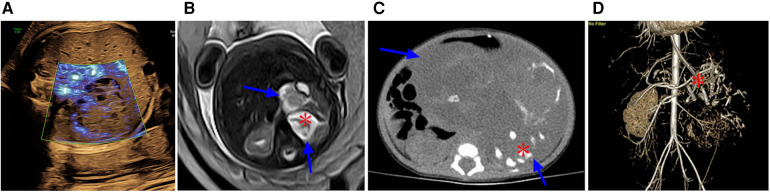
(A) At 29 weeks of gestation, a solid cystic mass with a clear boundary was observed in front of the fetal left kidney. Prenatal ultrasound indicated an adrenal solid cystic mass. (B) Image obtained at 29 weeks of gestation showing a parasitic fetus in the left upper abdomen. T2WI in the transverse view showed two interconnecting lesions in the left upper abdomen (blue arrow). The lesions in the rear were mainly cystic, and suspicious skeletal structures could be seen in the cyst (asterisk). (C,D) Images obtained six days after the birth of the fetus in image A and B. (C) Plain CT scan showed a significantly enlarged lesion with predominantly cystic components (blue arrows) and skeletal components (red asterisk) in the left upper abdomen; the lesion size was approximately four times larger than that at 29 weeks of gestation. (D) CT-enhanced 3D reconstruction with multiple long bone components in the left upper abdomen (red asterisk).
